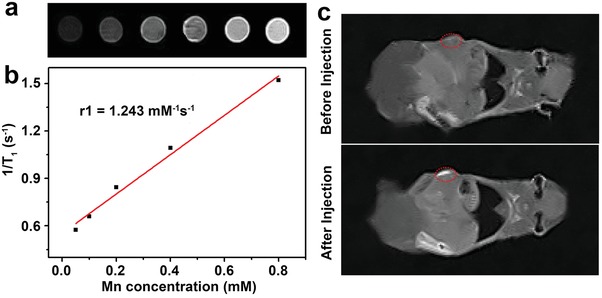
a) T1‐weighted MR images of Cu_2_
